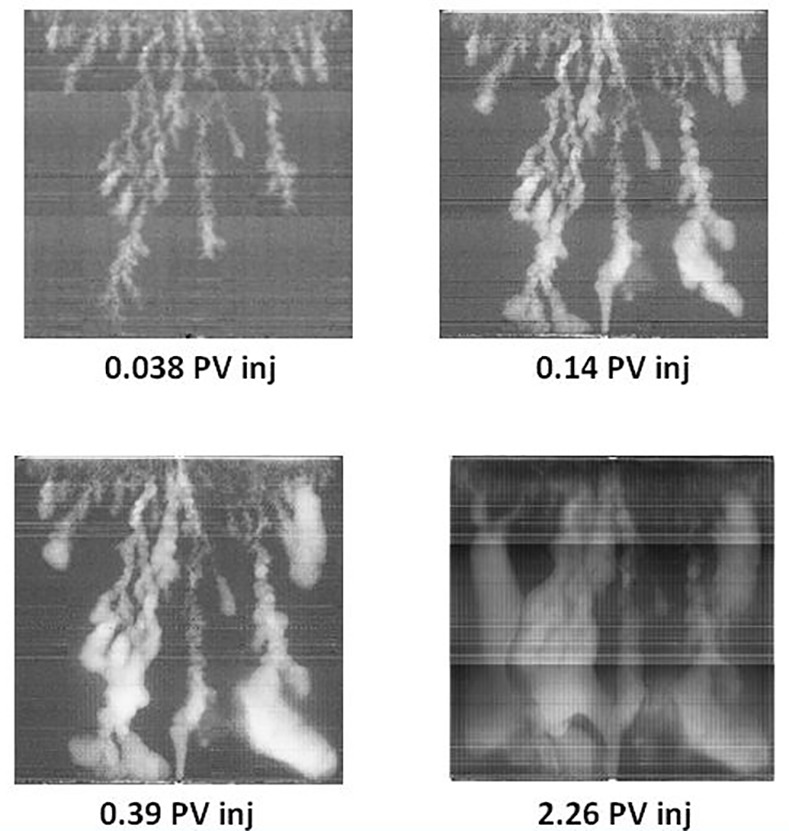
X-Ray images of saturation maps of E2000 experiment for different injected pore volumes [2]. The water (white) is injected from the bottom to displace the oil (black).
